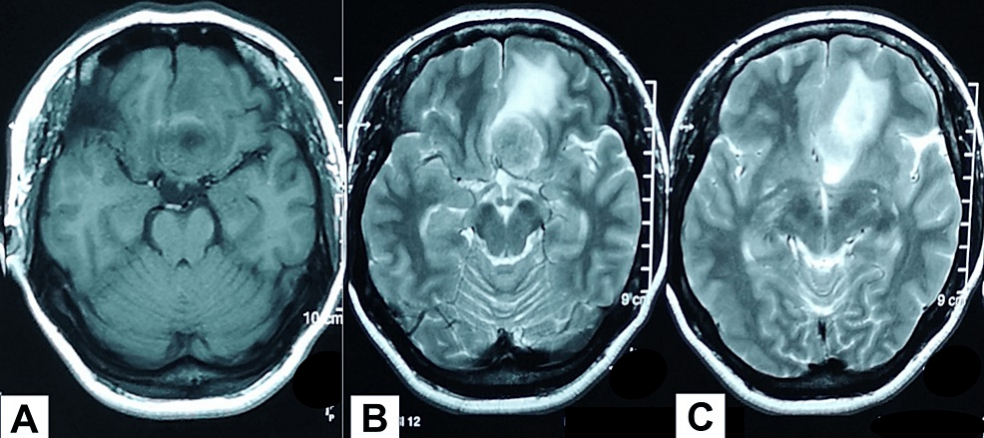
MRI shows a T1-isointense suprasellar lesion measuring 2.6 x 2.6 x 1.9 cm (A). The same lesion appeared hyperintense in T2 (B), with extension to the left anterior frontal lobe (C).
